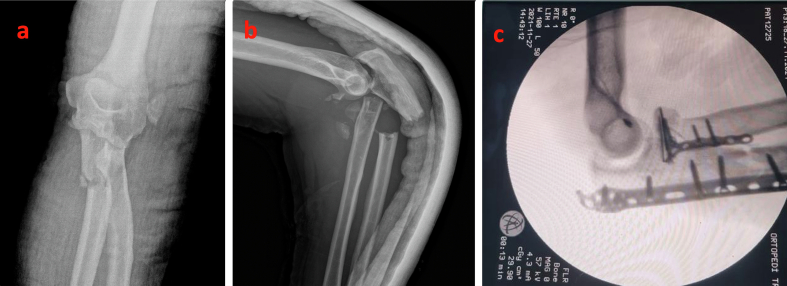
Elbow X-rays upon admission A) Anterior-posterior view. B) Lateral view. C) Intraoperative imaging.
